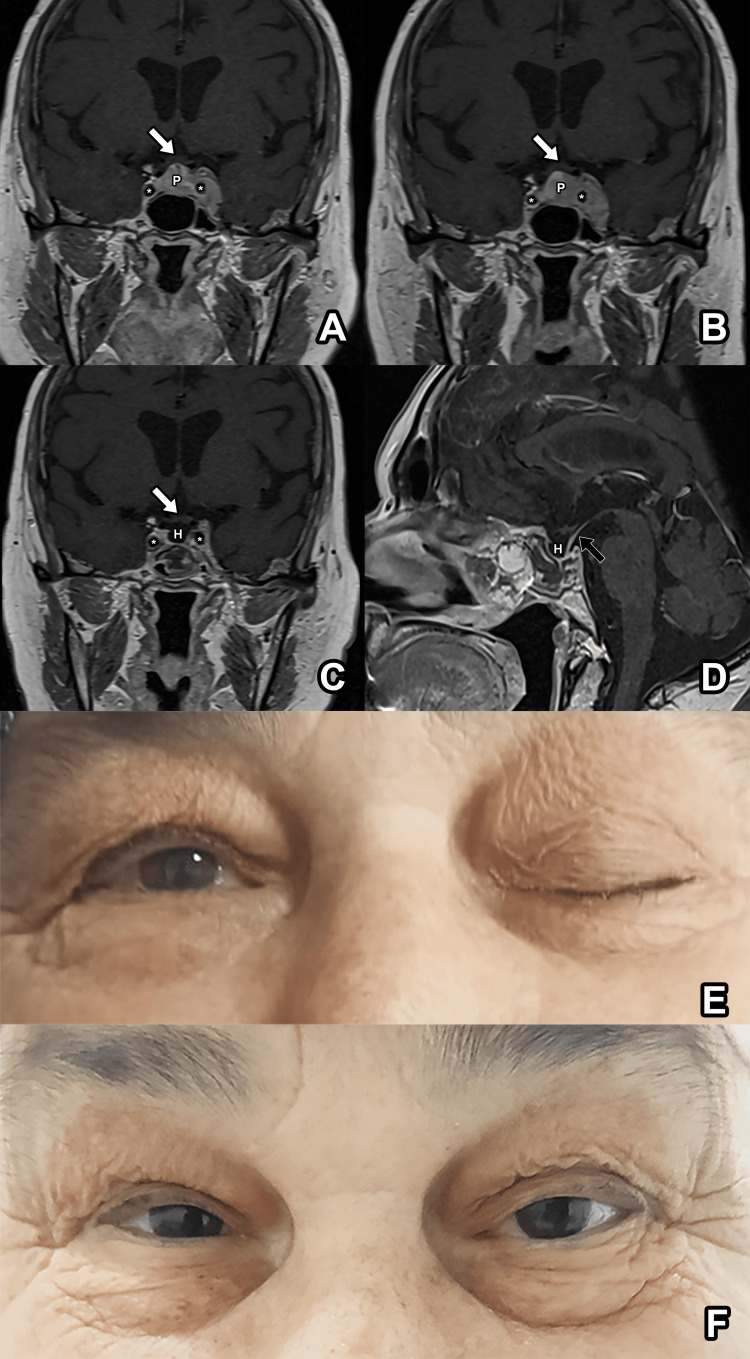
Pre- and postoperative MRI and ophthalmological examination of the patient. A) Initial coronal T1-weighted contrast-enhanced magnetic resonance imaging (MRI) scan reveals Knosp grade 4 pituitary lesion. B) Coronal T1-weighted contrast-enhanced MRI scan obtained a month after the initial MRI reveals that the tumor significantly increased in size (from 12.4 x 28.2 mm to 14.2 x 31.6 mm). Coronal (C) and sagittal (D) T1-weighted contrast-enhanced MRI scans obtained in the second postoperative month revealed almost complete disappearance of the tumor. E) Preoperative ophthalmological examination reveals total ophthalmoplegia, which completely resolved (F) in the second postoperative month. White arrow: optic chiasm, P: primary pituitary lymphoma, asterisk: internal carotid artery, H: hypophyseal fossa, black arrow: pituitary stalk.
